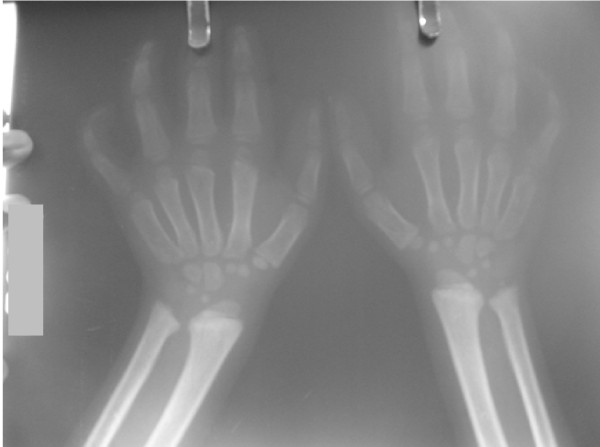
X-ray of the hands showing phalanges and metacarpals are widened with proximal tapering of metacarpals.
